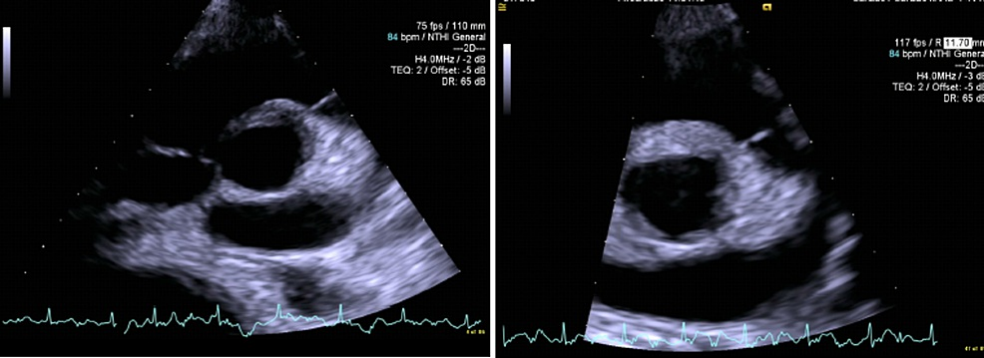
Echocardiogram showing complete resolution of the vegetation after antibiotic therapy
